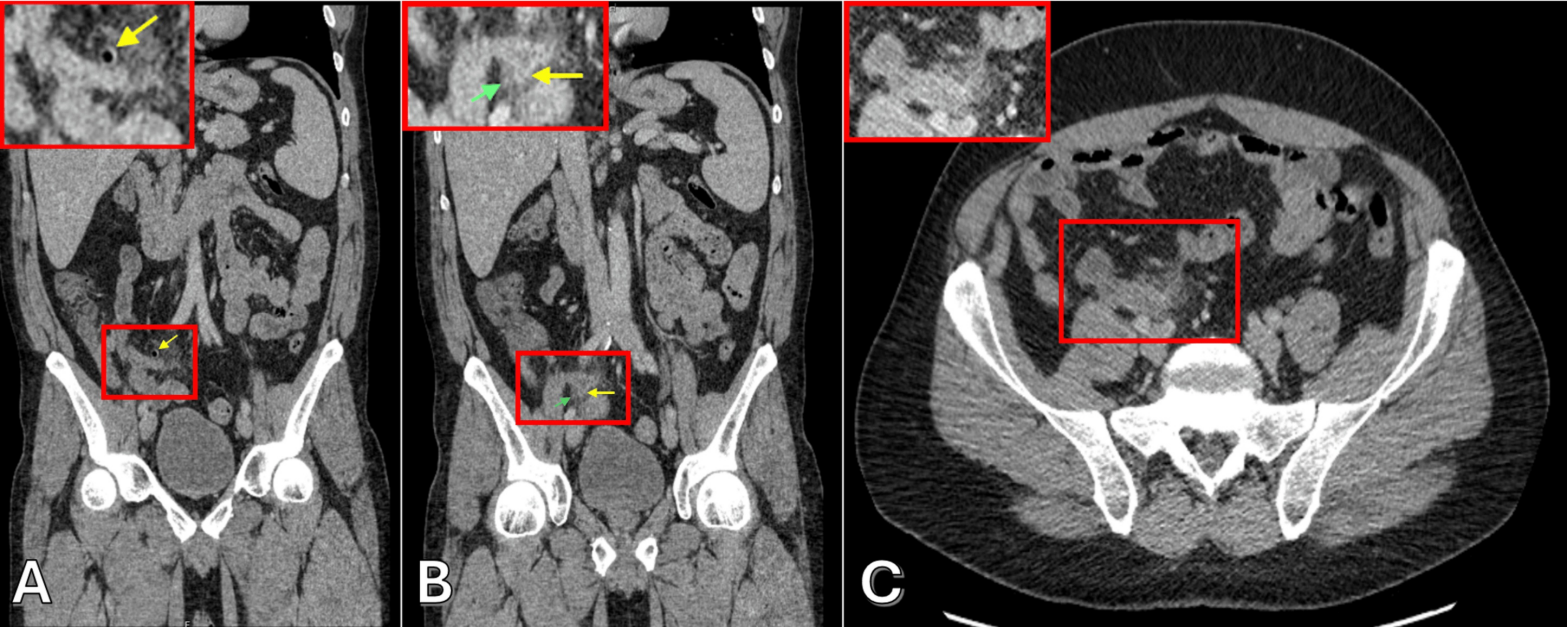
CT (computed tomography) scan images of appendiceal diverticulosis and diverticulitis. (A) Coronal view demonstrates the appendix (red box, with magnified insert) with gas micro-loculation (yellow arrow), a diverticular outpouching containing gas without inflammation. (B) Coronal image demonstrating a saccular appendix (red box, with magnified insert) with periappendiceal inflammatory changes (green arrow) and a fluid-filled diverticulum (yellow arrow), consistent with diverticulitis. (C) Axial image demonstrating a 1.4 cm thickened distal appendix with asymmetric periappendiceal fat stranding (red box, with magnified insert), further supporting the diagnosis of diverticulitis.
